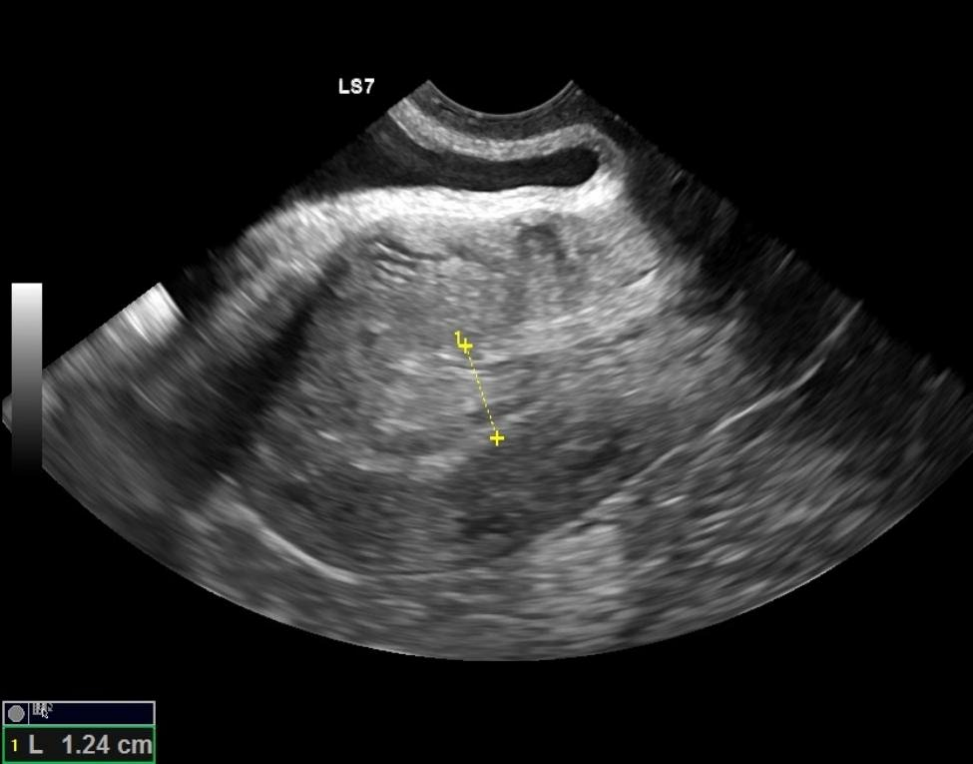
Transvaginal sagittal 2D ultrasound image one week after medication abortion. Ultrasound revealed expected thickened endometrial complex and absence of gestational sac, which was consistent with a medication abortion that was complete. No obvious retained products of conception were present
